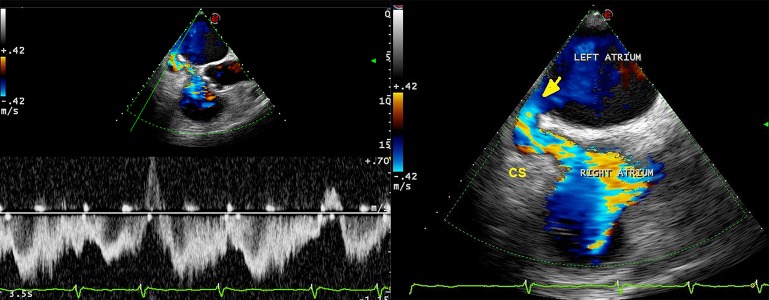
Transesophageal echocardiogram; left: Pulsed wave Doppler revealed flow through LA to CS. Right: contrast injection showed bubble passage through right atrium and CS to LA, demonstrating atrial shunt (yellow arrow) Abbreviations: CS: Coronary Sinus; LA: Left Atrium
